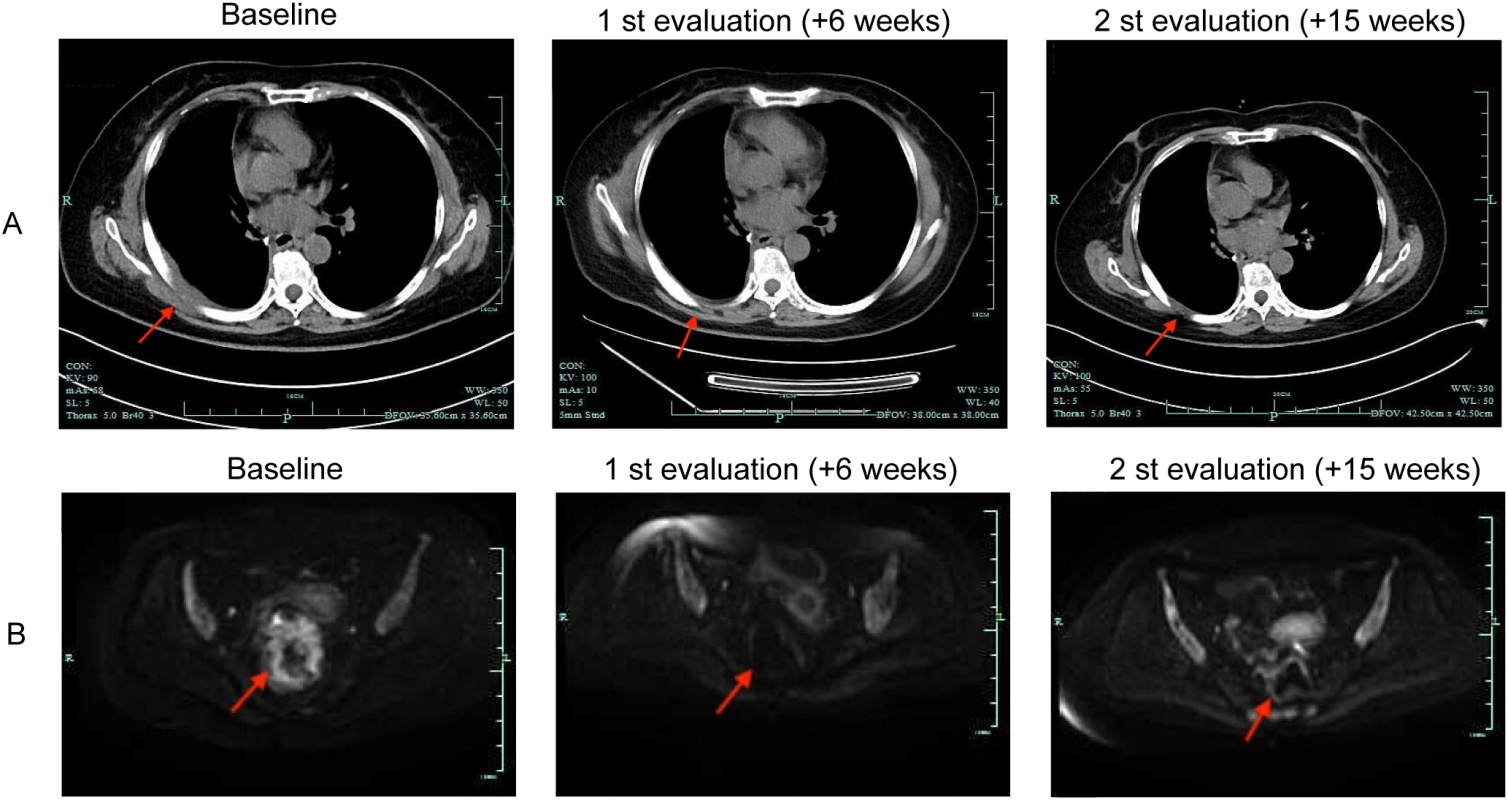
Radiologic images before and after iparomlimab and tuvonralimab. (A) Representative chest computed tomography (CT) images. (B) Representative pelvic enhanced magnetic resonance imaging (MRI) images.
